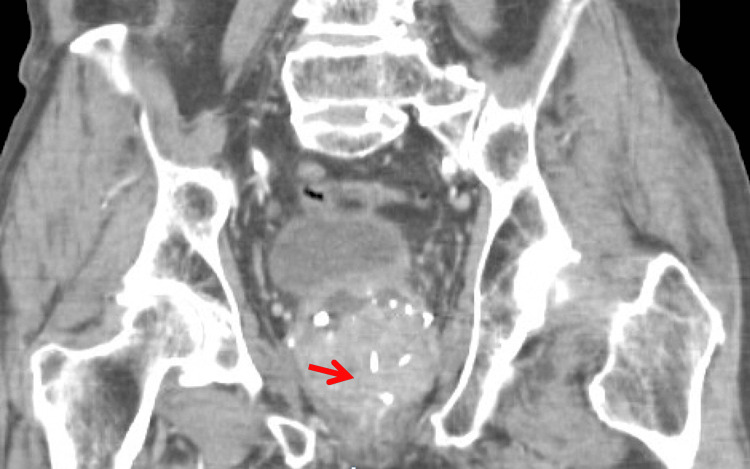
CT abdomen and pelvis, coronal view. Enlarged prostate gland (arrow) contributing to bladder outlet obstruction
